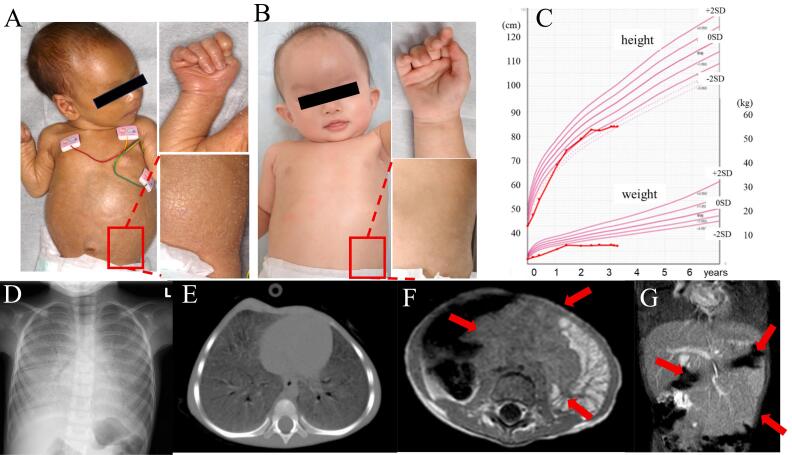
Physical and imaging findings of the patient. Photographs of the patient's external body at birth (Fig. A) and at nine months (Fig. B). She exhibited a collodion skin at birth. After enzyme replacement therapy, the collodion skin improved. Fig. C shows her growth curve. Decreased growth rate and poor weight gain was observed during two years after birth. Fig. D, E, F, and G show Chest X-ray, computed tomography (CT) image, and abdominal magnetic resonance imaging (MRI) image, respectively. These Figures demonstrate ground-glass opacity and enlarged mesenteric lymph nodes at the age of two years eight months.
